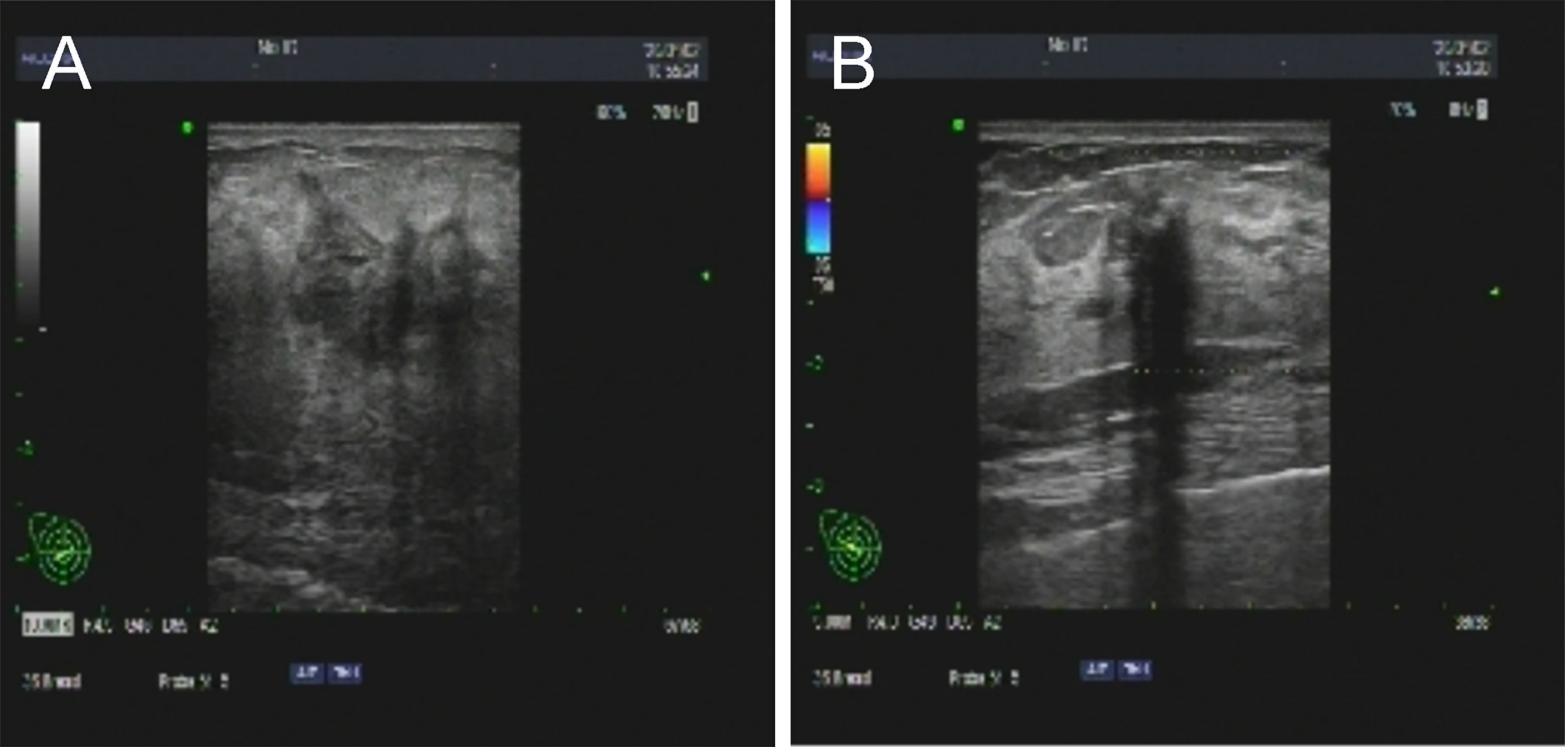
The ultrasound revealed 2 abnormal-echoic lesions in the left (A) and right (B) breast on which core needle biopsy was subsequently performed.
